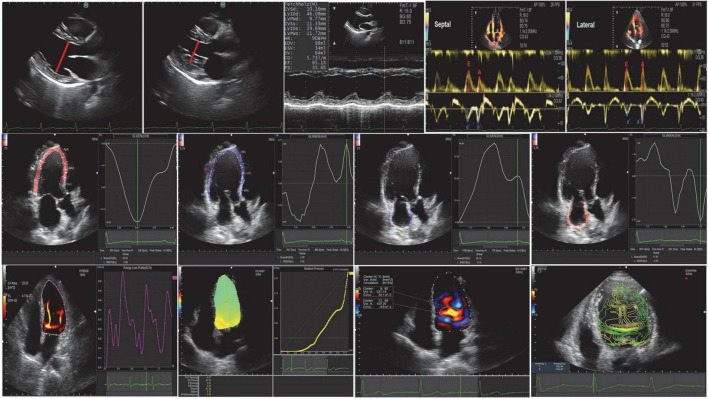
Conventional echocardiography, speckle-tracking echocardiography, and vortex flow mapping echocardiography.
